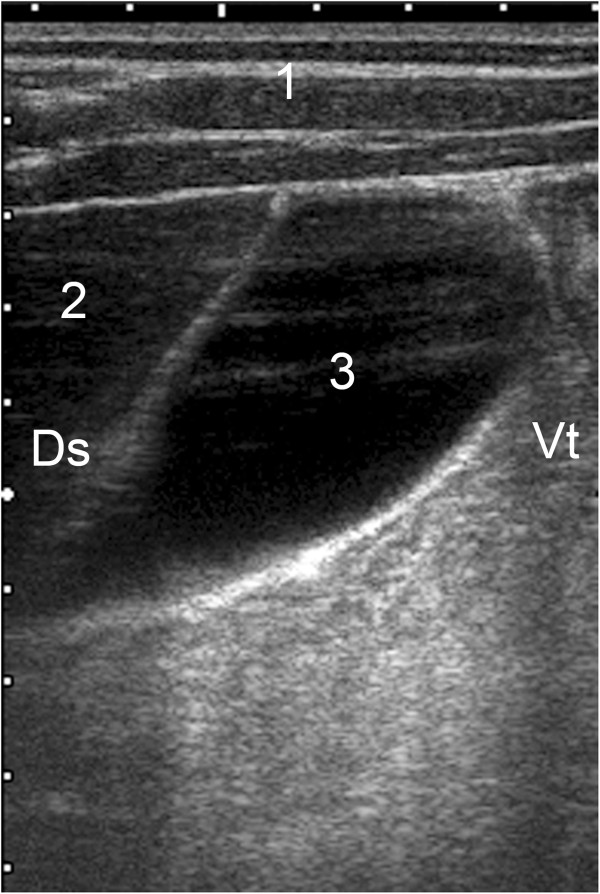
Ultrasonogram of the gallbladder. Ultrasonogram of the gallbladder viewed from the right in a 62-day-old Holstein-Friesian calf. 1 Abdominal wall, 2 Liver, 3 Gallbladder, Ds Dorsal, Vt Ventral. (depth of the image = 9 cm).
